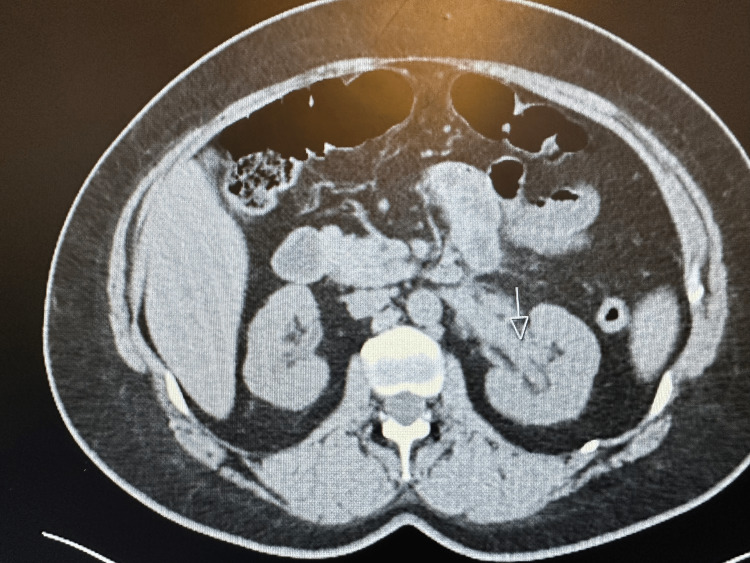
CT-Abdomen showing the left renal pelvis and proximal ureteral thickening.
